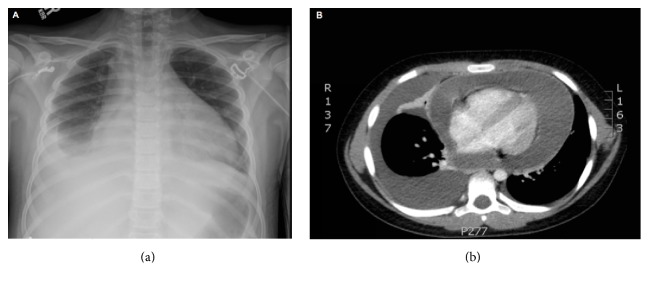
(a) Admission chest X-ray demonstrating enlarged cardiac silhouette with associated right-sided pleural effusion. (b) Computed tomography showing large circumferential pericardial effusion and associated pleural effusion.
